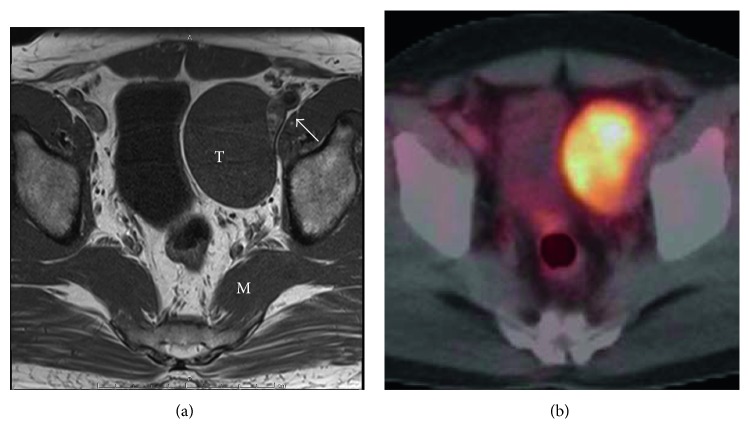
(a) Magnetic resonance imaging showed that the tumor (T) was isointense with muscle (M) in the T1-weighted image. The tumor adjoins the left external iliac vein (arrow). (b) Positron-emission computed tomography scan showed abnormal integration at the tumor site.
